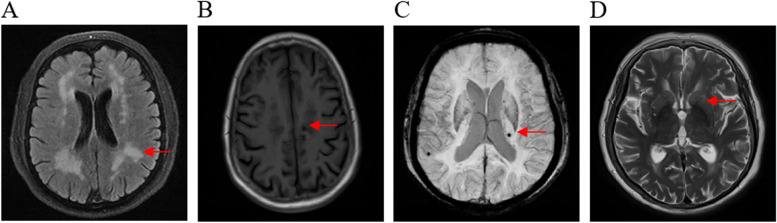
MRI manifestations of cerebral small vessel disease
